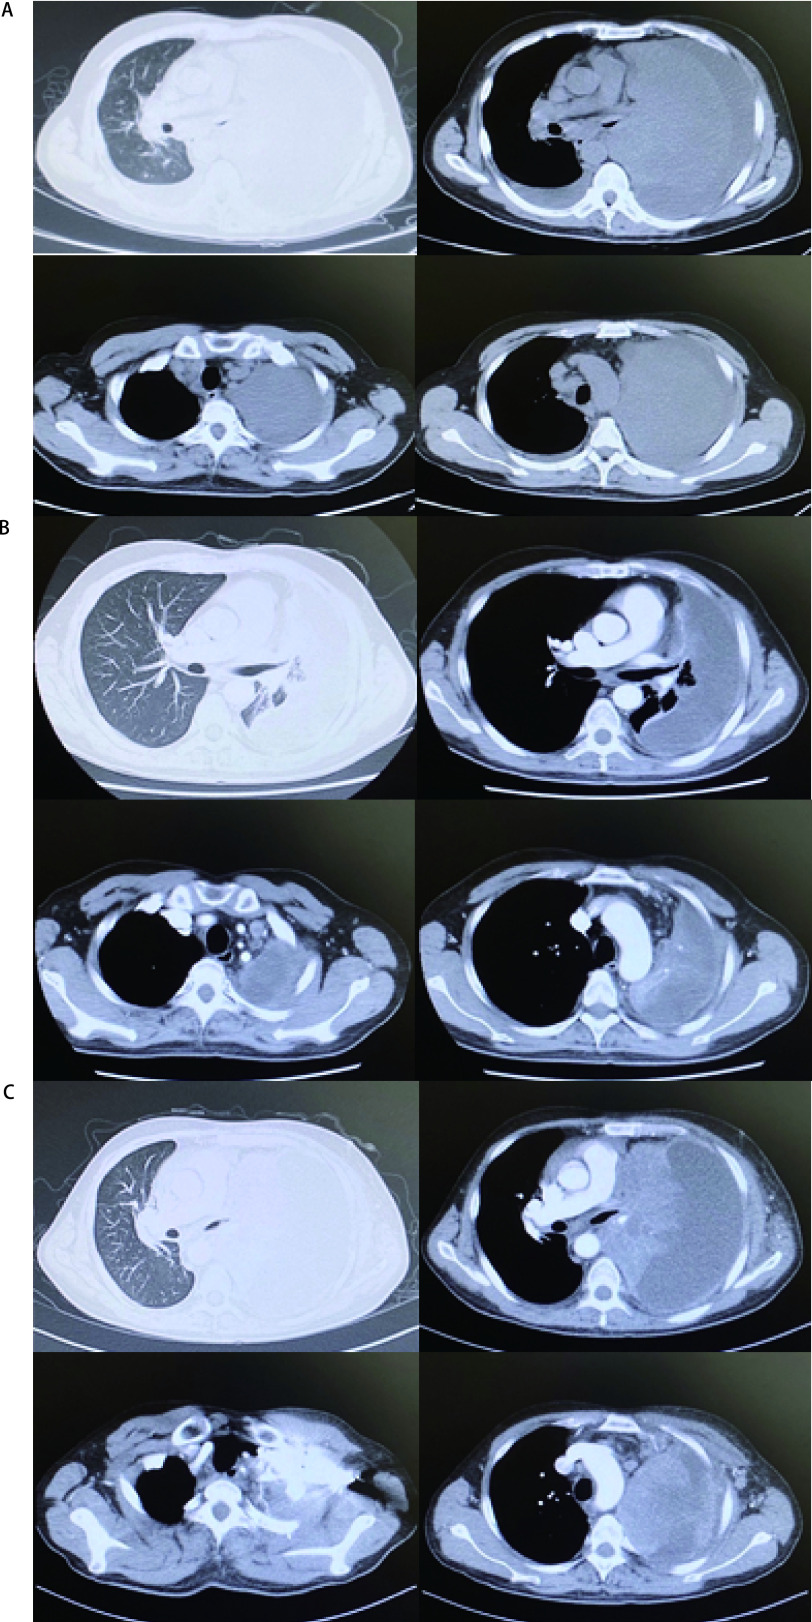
胸部CT对比。A：患者基线影像学检查（2021年4月26日）：可见左主支气管截断，左肺致密实变影（肿瘤病灶与肺不张分界不清），纵隔多发淋巴结肿大；B：2021年7月14日疗效评价：左肺肿块与左上肺分界不清，范围较前缩小，左上肺较前复张；纵隔及右侧肺门多发肿大淋巴结较前缩小；C：患者2021年9月15日疗效评价：左肺肿块，较前范围明显增大，伴左侧全肺不张，纵隔6区淋巴结较前明显增大。 Chest CT contrast. A: Baseline imaging examination of patient (April 26, 2021): truncation of the left main bronchus, dense consolidation of the left lung (unclear boundary between tumor and atelectasis) and multiple mediastinal lymph gland can be seen; B: Effect evaluation on July 14, 2021: the boundary between the left lung mass and the left upper lung is unclear, the scope is narrower than before, and the left upper lung is more dilated than before; the mediastinum and the right hilum of the lung were multiple and the lymph nodes were smaller than before; C: Effect of evaluation on September 15, 2021: the left lung mass is significantly larger than before, accompanied by left total atelectasis, and para-aortic lymph nodes was significantly larger than before. CT: computed tomography.
